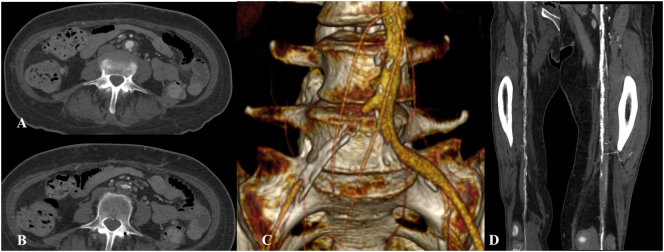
Preoperative CT-angiography showing significant atherosclerotic aortic disease (A and B), right common iliac artery (C) and femoral popliteal axes.
